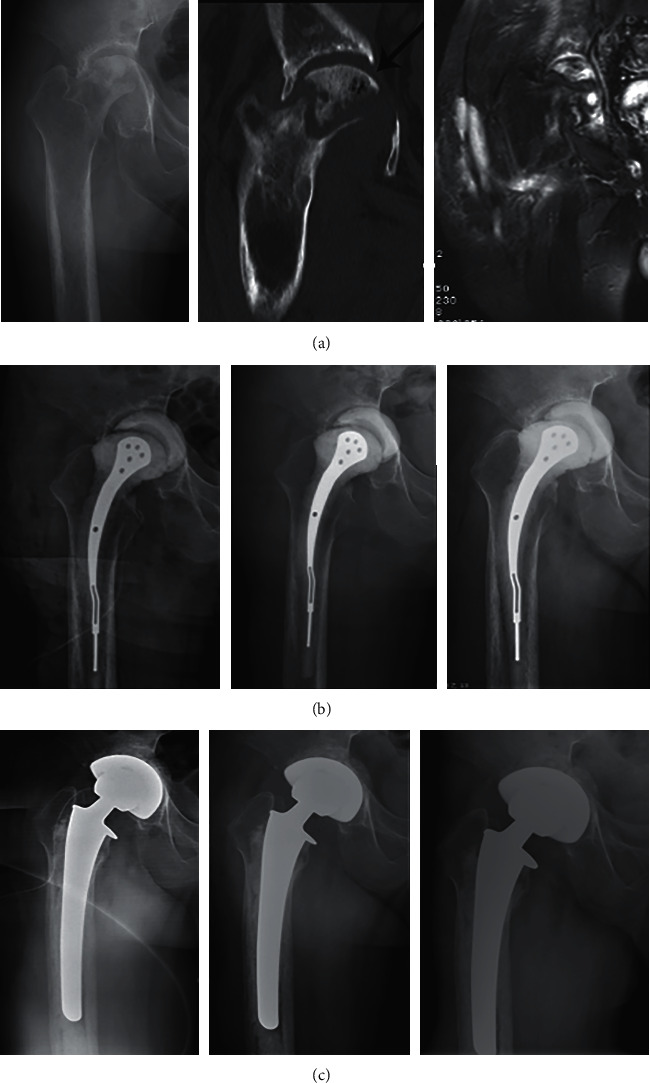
Radiographs of a 54-year-old man with right hip joint pain and severe limitation of movement. (a) Anteroposterior X-ray and coronal CT images of the pelvis showed that the right acetabulum was invaginated and the acetabulum wall was damaged (arrow). Coronal MRI of the pelvis showed inflammatory changes in the right hip joint. (b) The postoperative imaging of femoral head resection with antibiotic cement implantation at the same day, 1 month, and 6 months, respectively. (c) The imaging findings after THA at 0 days, 1 month, and 12 months, respectively.
